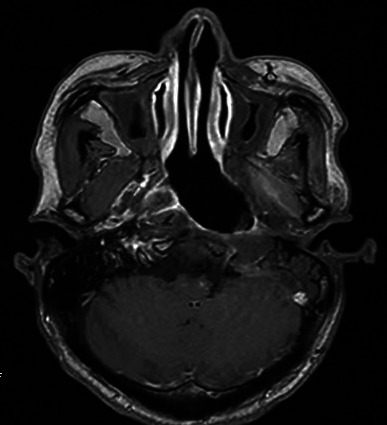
Patient's imaging results 8 months after the fourth IA. Enhanced MRI revealed that there were no signs of tumors
